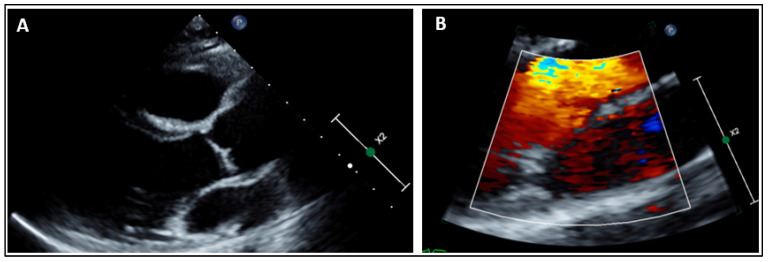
Parasternal long axis view ruling out any mitral valve prolapse (A). Mitral valve leaflets are thin without chordae elongation. Parasternal long axis view with color Doppler ruling out any mitral regurgitation in systole (B).
